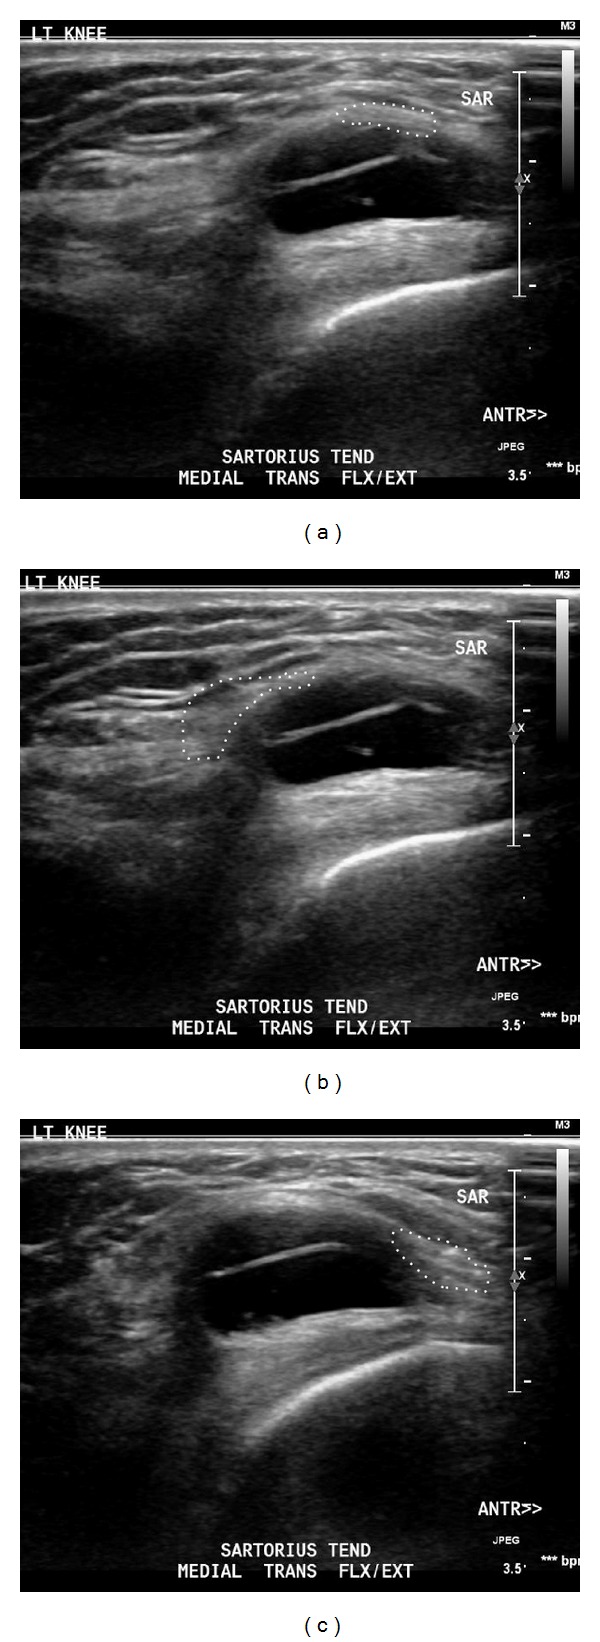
A series of dynamic ultrasound images taken in knee flexion/extension cycle, showing position of distal sartorius musculotendinous junction passing over parameniscal cyst; a palpable “snapping sensation” was felt through the transducer: (a) neutral extension; (b) in semiflexion; (c) extension with active quadriceps contraction; note that the tendon of sartorius passes forwards.
